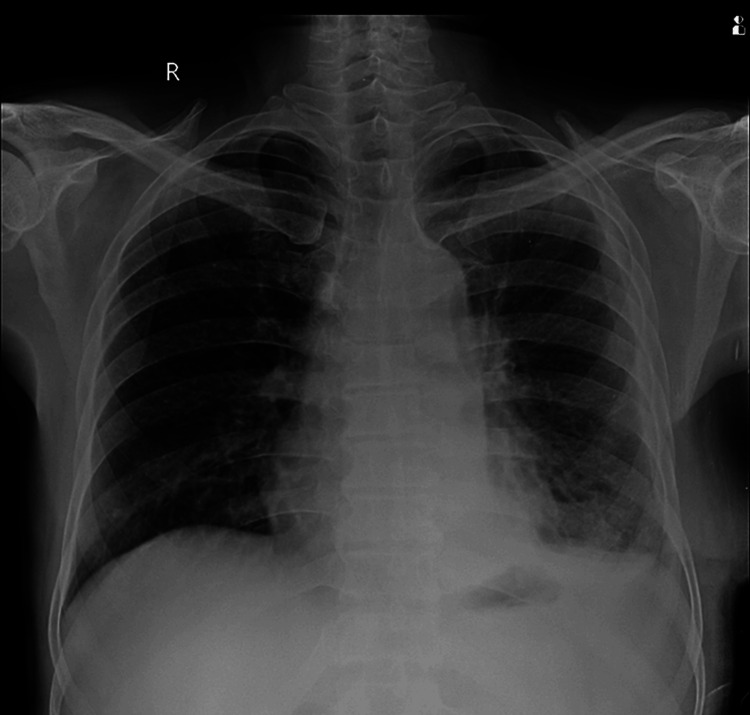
Chest X-ray post-operative day 14
